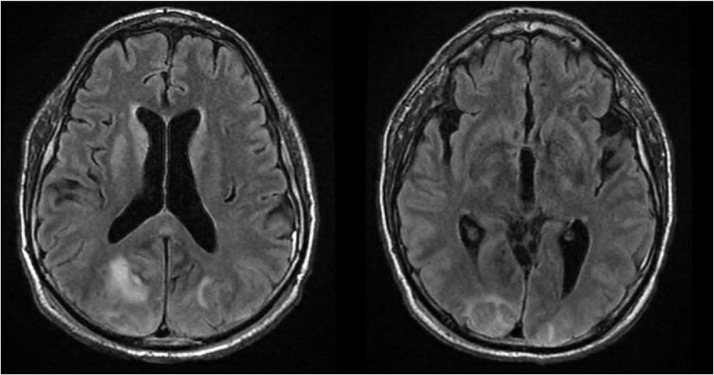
MRI showing area of ischemia in right occipital lobe possibly due to right vertebral artery dissection.
